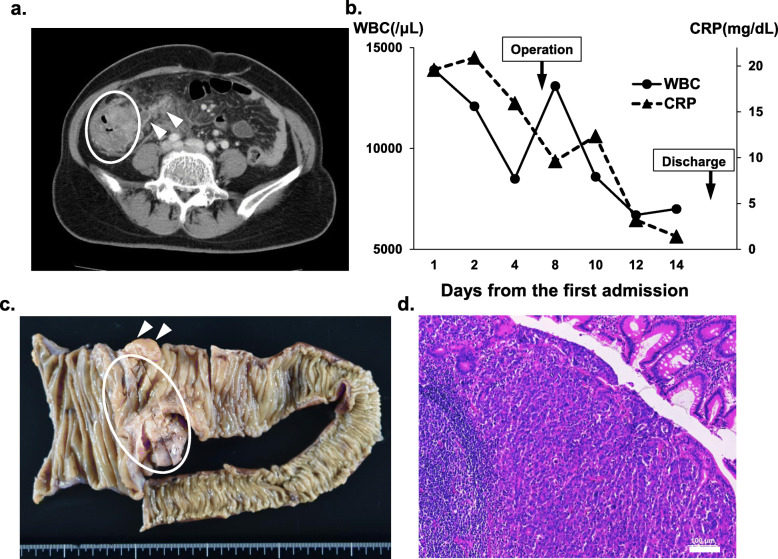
a CT findings: CT revealed acute appendicitis (arrow heads) with abdominal abscess and irregular wall thickness of the cecum (circle). b The clinical course of this patient: she underwent interval operation at day 7 and left the hospital at postoperative day 9. c A macro-finding of the resected organ: the arrow heads indicate the appendix. The oval indicates the tumor lesion. d Pathological examination: pathological features revealed poorly differentiated adenocarcinoma
